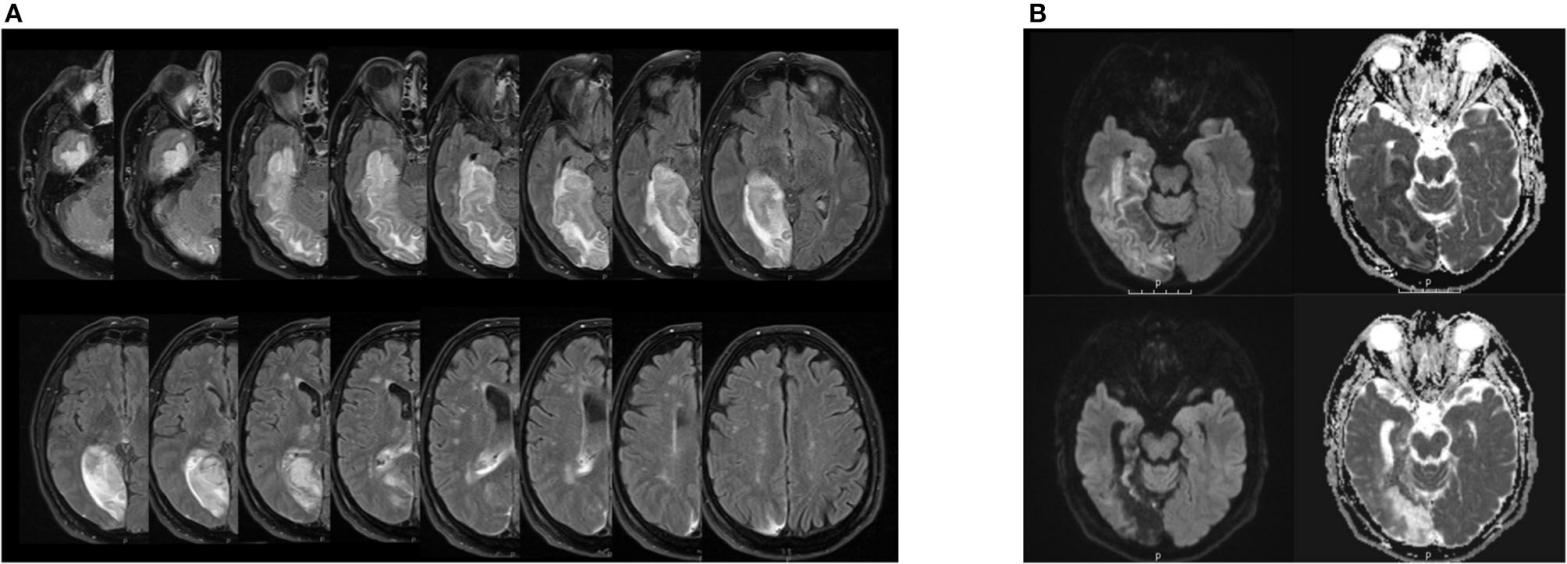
(A) Lesion extension in patient EP in the follow-up MRI after acute treatment. Right hemisphere is on the left side of the each image respectively. FLAIR sequence from a cerebral magnetic resonance imaging, 3 Tesla, with on the upper left side of the image the inferior and the lower side of the image the upper bound of lesion estension. The hyperintense signal displays the demarcated subacute ischemic and hemorrhagic transformations in the right PCA-territory encompassing the right thalamus, cuneus, hippocampus, gyrus parahippocampalis and the precuneus as well as the right medial and posterolateral thalamus. (B) Hippocampal lesion in patient EP in the follow-up MRI after acute treatment (upper images) and 3 months after symptom onset (lower images). Right hemisphere is on the left side of each image respectively. DWI sequence from a cerebral magnetic resonance imaging, 3 Tesla, on the left side b 1,000 and the ride side the ADC map. On the upper images, the diffusion restriction encompasses the hippocampus, the gyrus parahippcampalis and the temporo-occipital lobes depicted. Note the cortical diffusion restriction of the vental hippocampus. On the lower pictures 3 months after symptom onset, we find the post-ischemic parenchymal lesion of the formerly diffusion restricted regions with evacuo configurartion of the temporal ventricle and loco regional subarachnoid space.
